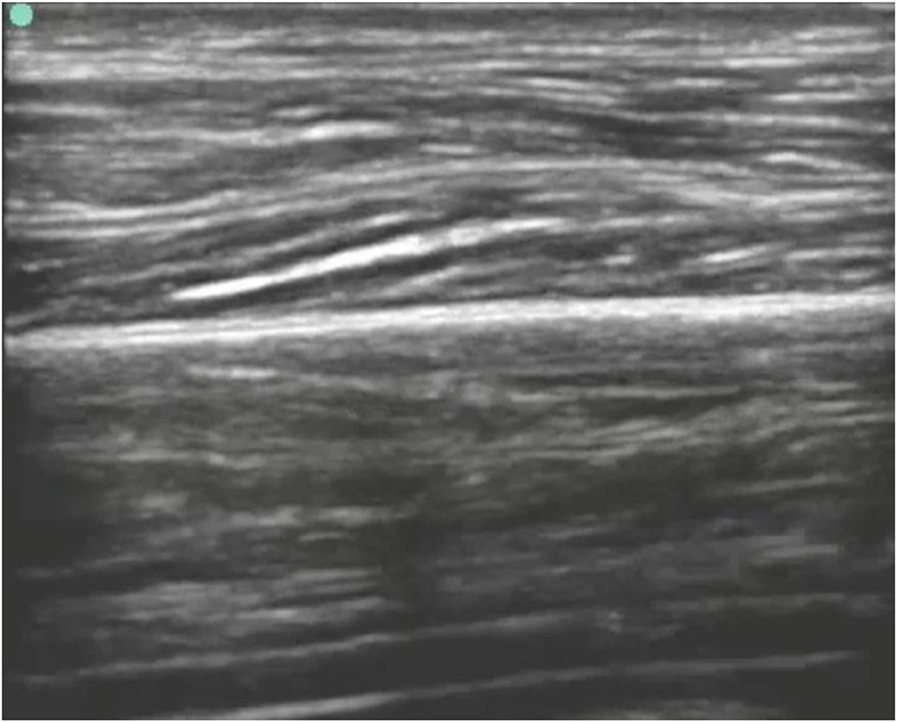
ED bedside ultrasonogram of a normal quadriceps muscle.
